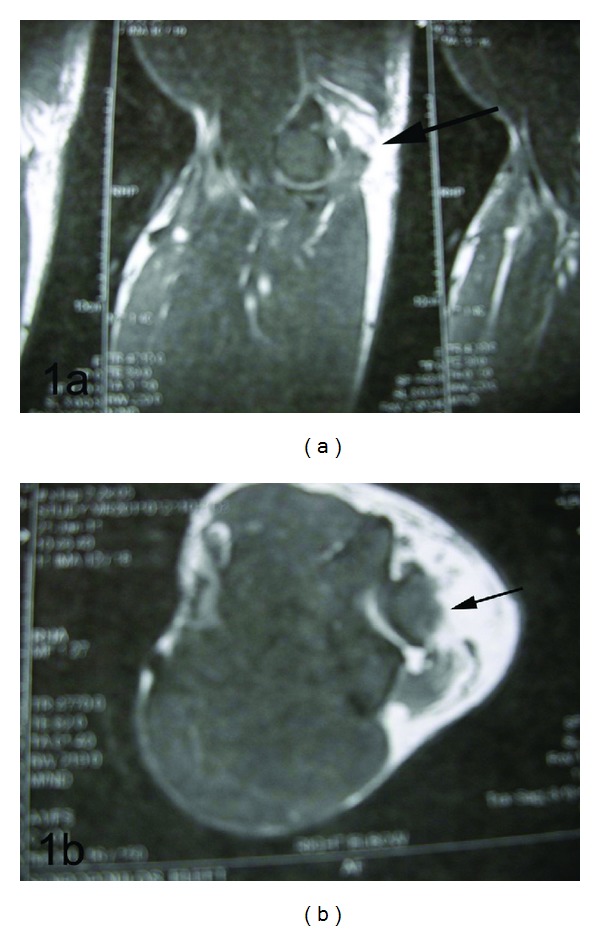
Preoperative MRI (scanned from hard copy) with arrows indicating the complete distal triceps tendon rupture at its insertion at the olecranon in a sagittal (a) and transverse (b) view.
